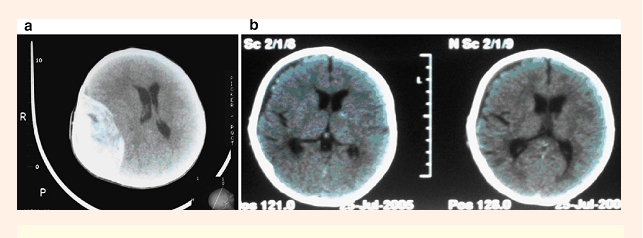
Preoperative(1a) and postoperative(1b) head CT scans in a 5–month–old infant with EDH
